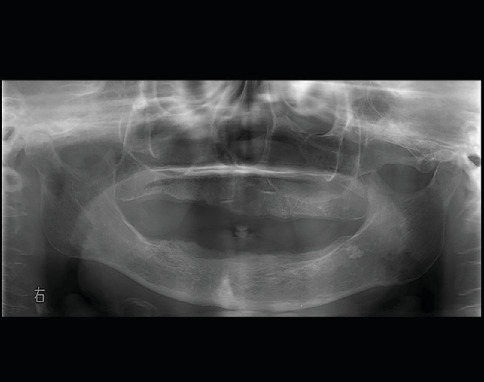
Panoramic radiography at first examination. No cysts, tumors, or structural changes are apparent in the maxilla or mandible.
